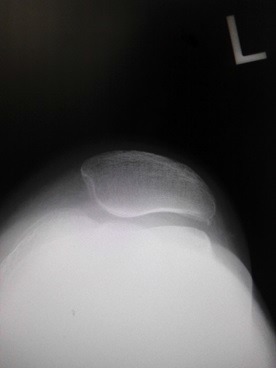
Roentgenogram taken prior to the injury shows no lateral patellar subluxation.
